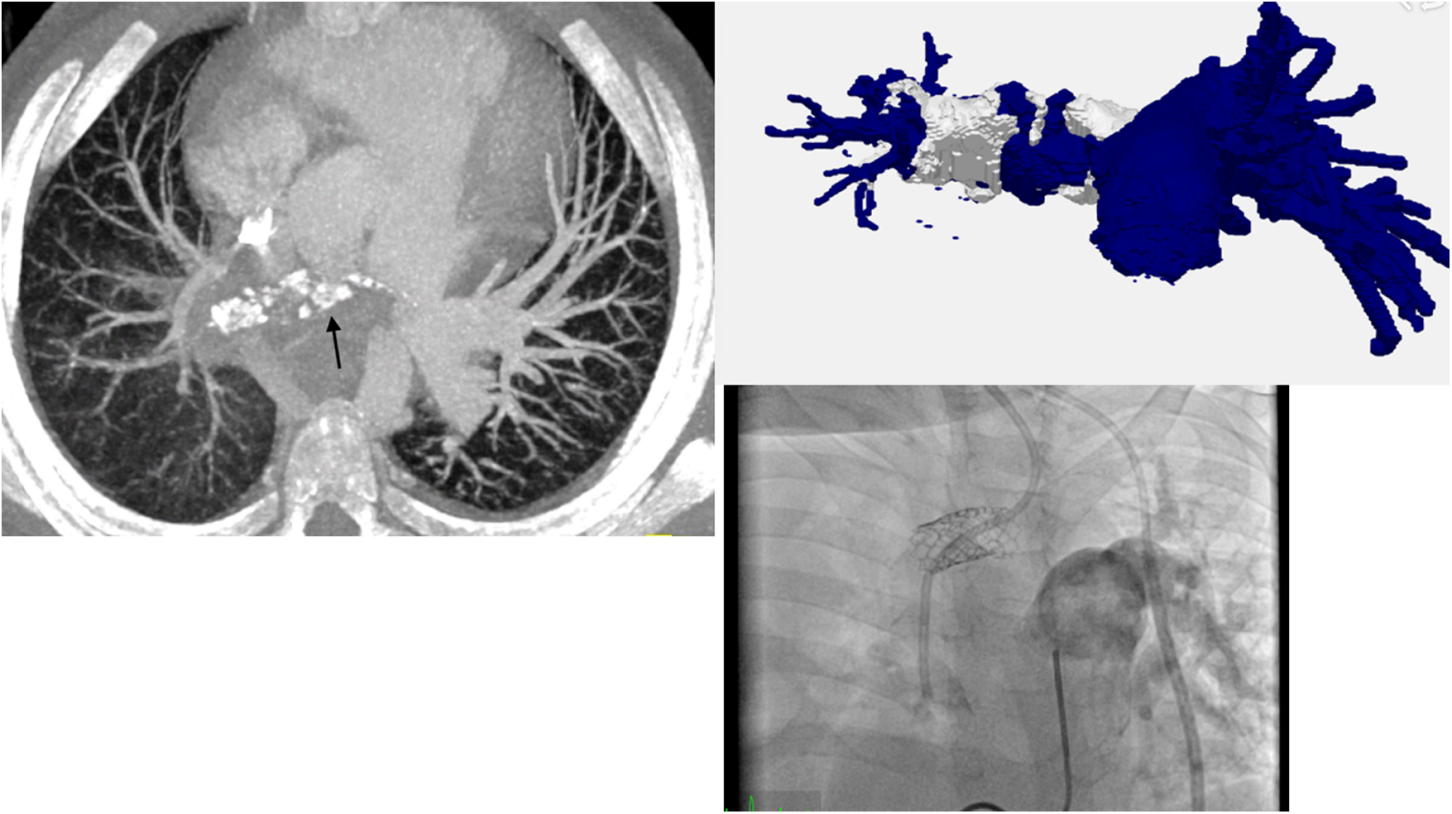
CT-angiography, 3D-reconstruction and pulmonary angiogram showing subtotal obstruction of the right pulmonary artery (patient 1).
